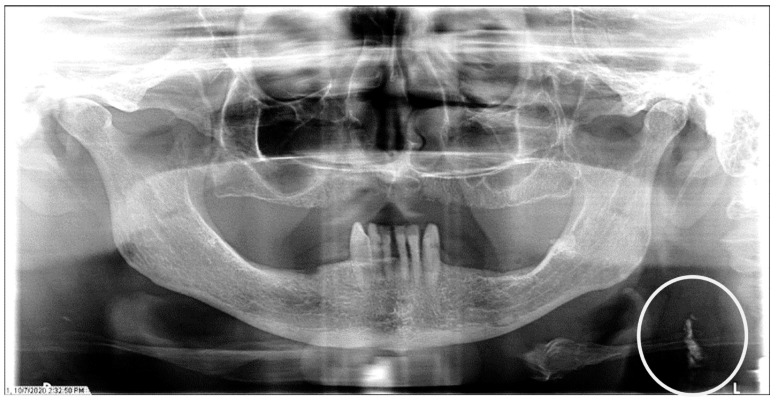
The panoramic radiograph of a 77-year-old female patient with a medical history of hypertension, hyperlipidemia, diabetes mellitus, and coronary artery disease showing a unilateral left carotid artery calcification (CAC) during a comprehensive dental examination. The CAC is encircled by a white line.
